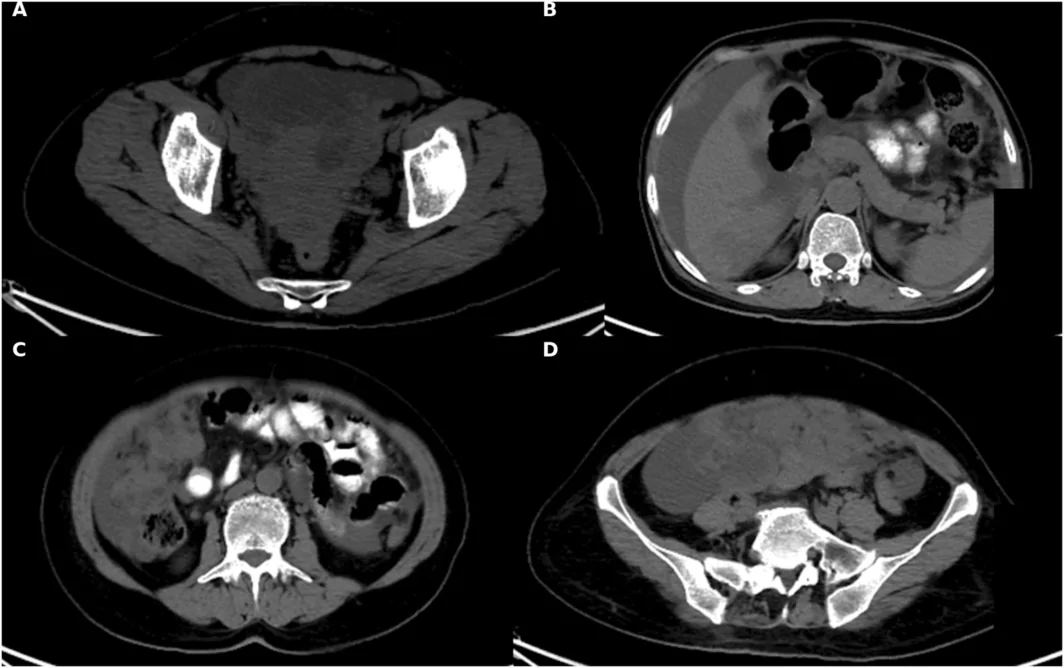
PET‐CT on August 22, 2024 (A–D). Baseline PET‐CT displays hypermetabolic bilateral adnexal lesions, diffuse peritoneal and omental implants, and massive ascites consistent with peritoneal carcinomatosis.
